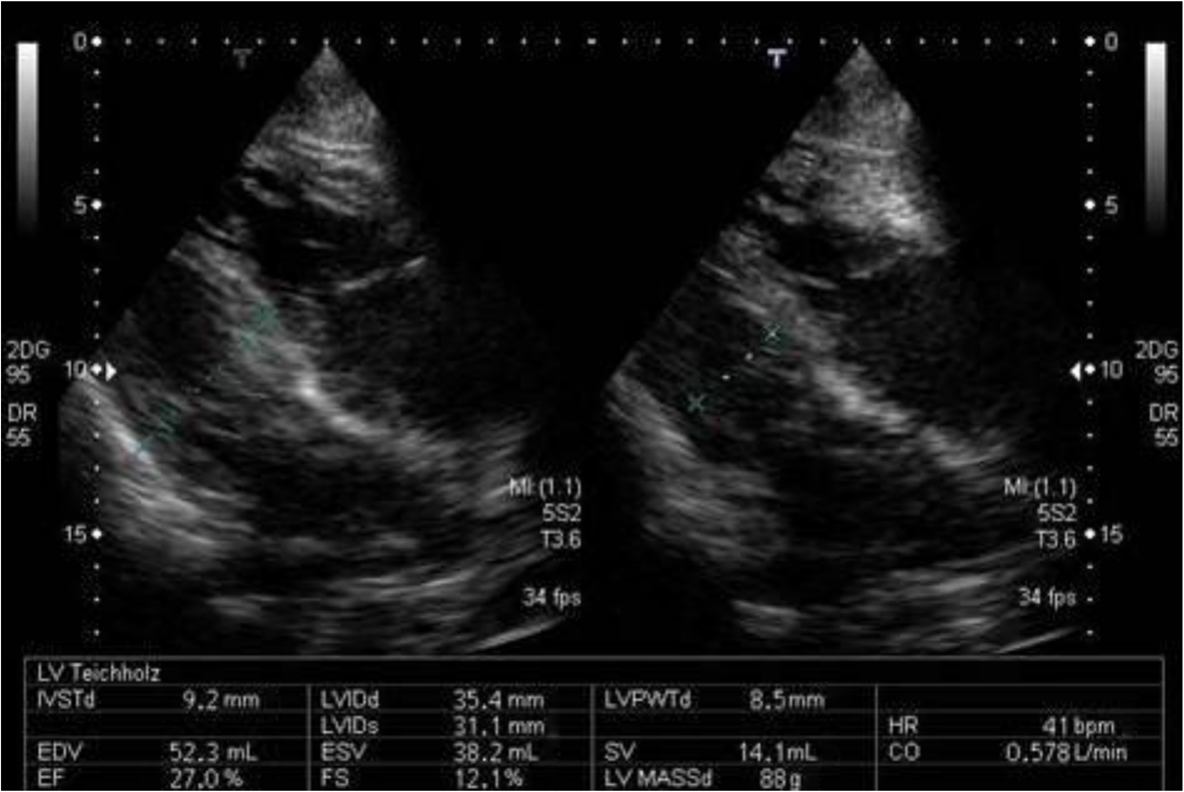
Echocardiogram of long axis view.
